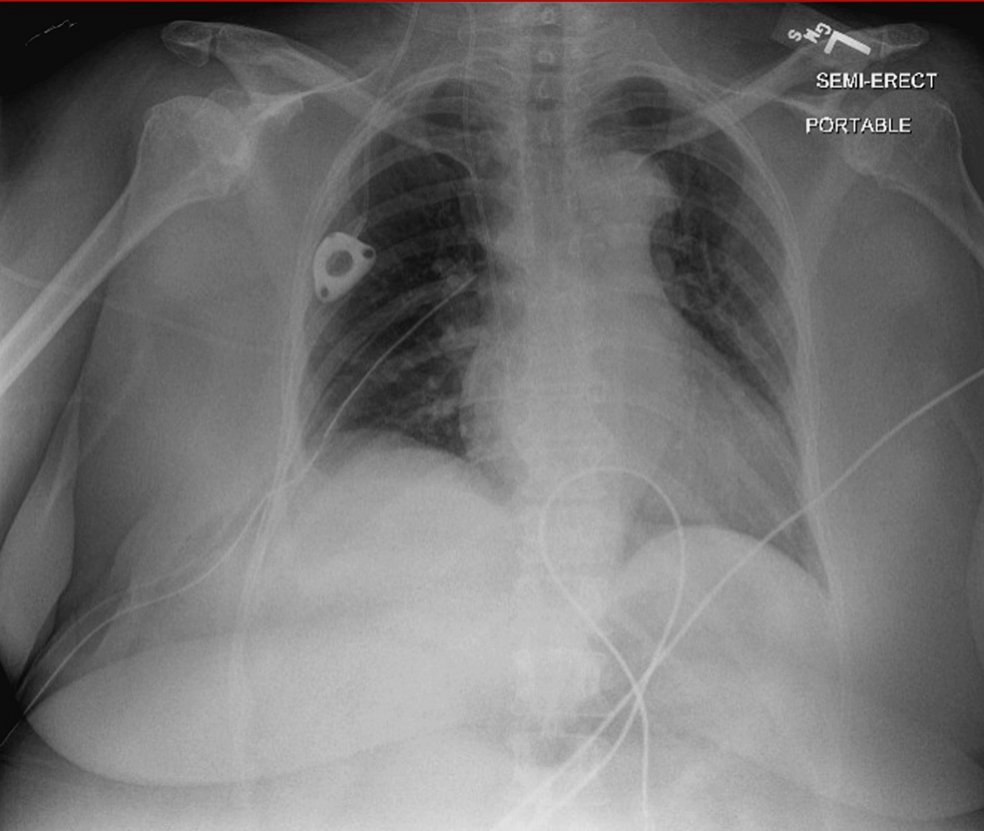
Post VATS chest radiograph VATS: Video-assisted thoracoscopic surgery The image is showing interval placement of the right-sided chest tube with complete resolution of right-sided pleural effusion.
